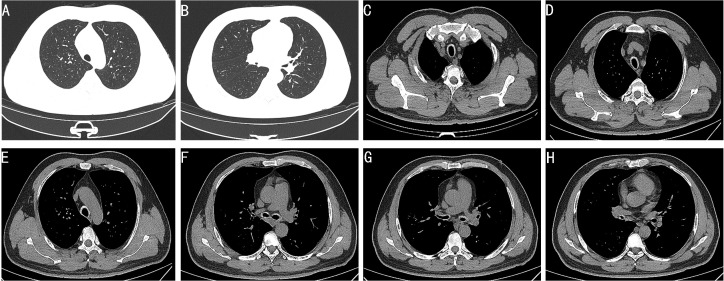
Computed tomography manifestations of airway involvement in relapsing polychondritis (RP). Images 1(A–B) show the lung window of patients with RP, with no significant abnormal findings. Images 1(C–H) show the mediastinal window, in which diffuse circumferential thickening of the trachea, left and right main bronchi, and right middle segment bronchus is observed, accompanied by localized calcification of the airway cartilage.
